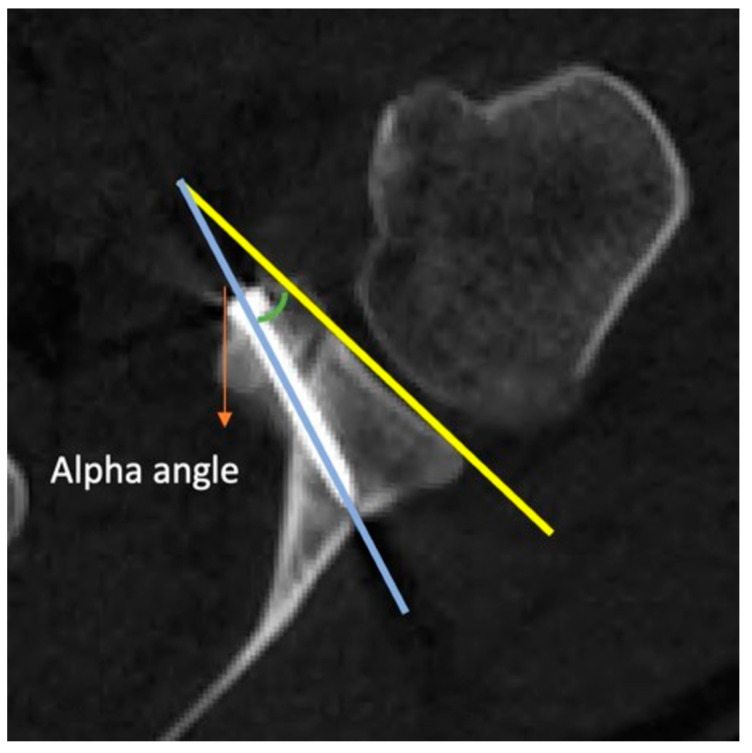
Measurement of the alpha angle on postoperative CT.
